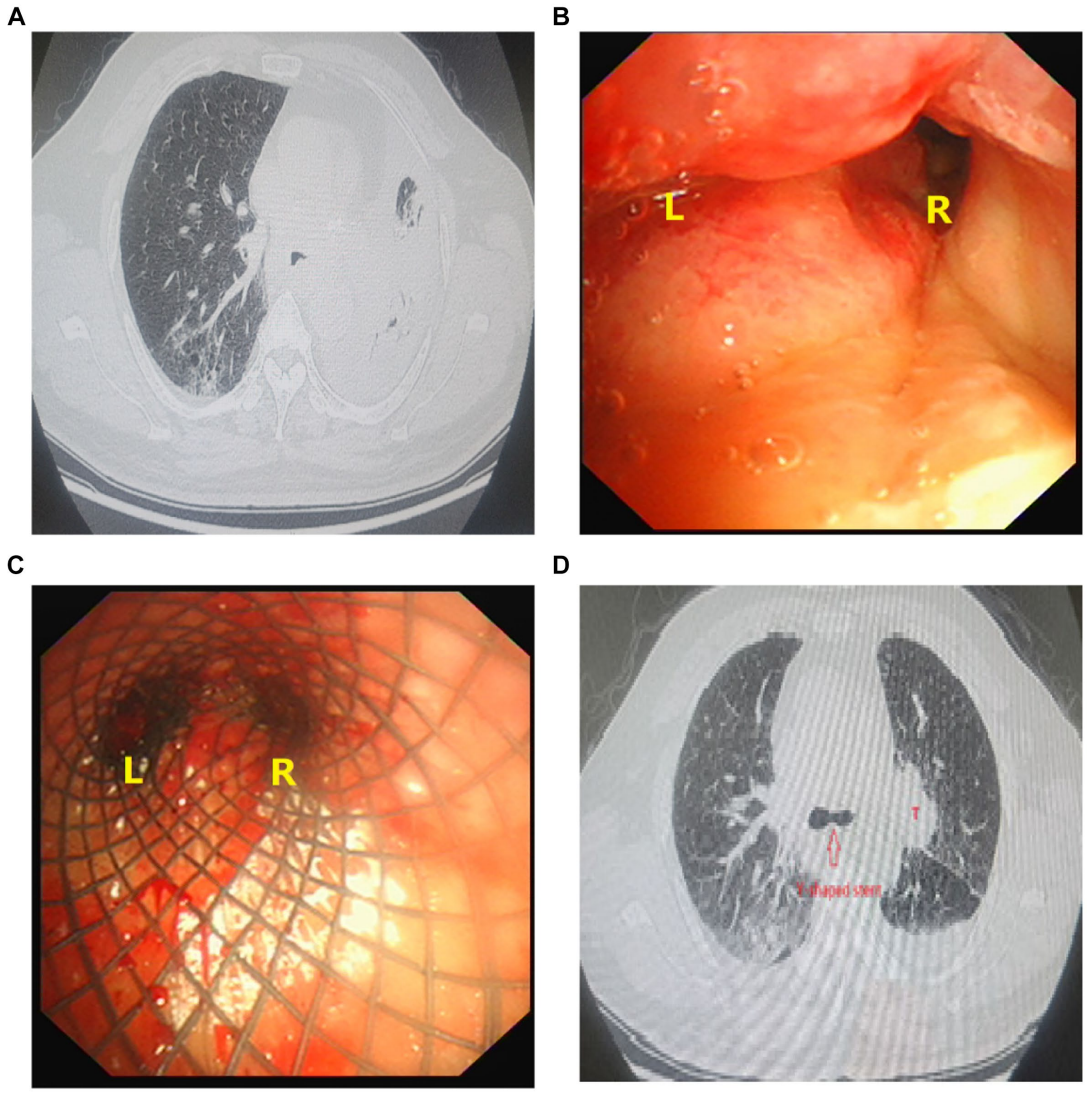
(A) Chest CT shows severe airway stenosis with left lung atelectasis. (B) Bronchoscopy shows complete obstruction of the left bronchus (L) due to tumor involving, right main bronchus (R) also involving by tumor with only about a quarter of the diameter remaining. (C) Y-shaped self-expanding metal covered stent was implanted within the patient’s airway. (D) Y-shaped self-expanding metal covered stent was implanted within the airway (), rustling in fully recover from left lung atelectasis. A tumor with a diameter of 5 cm in the left hilum (T).
